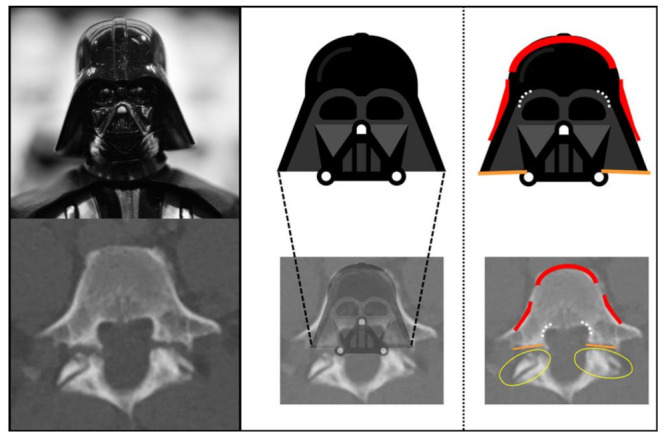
Typical appearance characteristics of Darth Vader and overlap with imaging features of spondylolysis in computed tomography.
